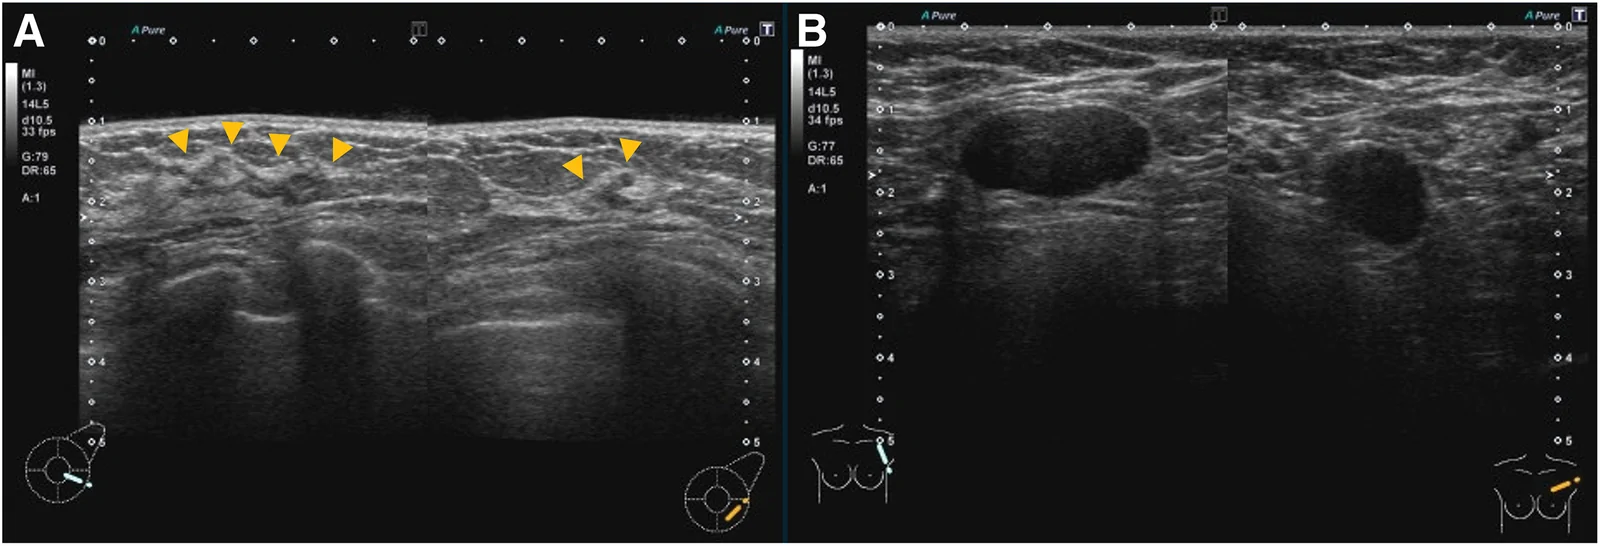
US findings. (A) Breast ultrasonography showed an indistinct hypoechoic lesion measuring 20 × 9 mm in the lower outer quadrant of the left breast (orange arrowheads). (B) Enlarged lymph nodes were detected in the axilla.
